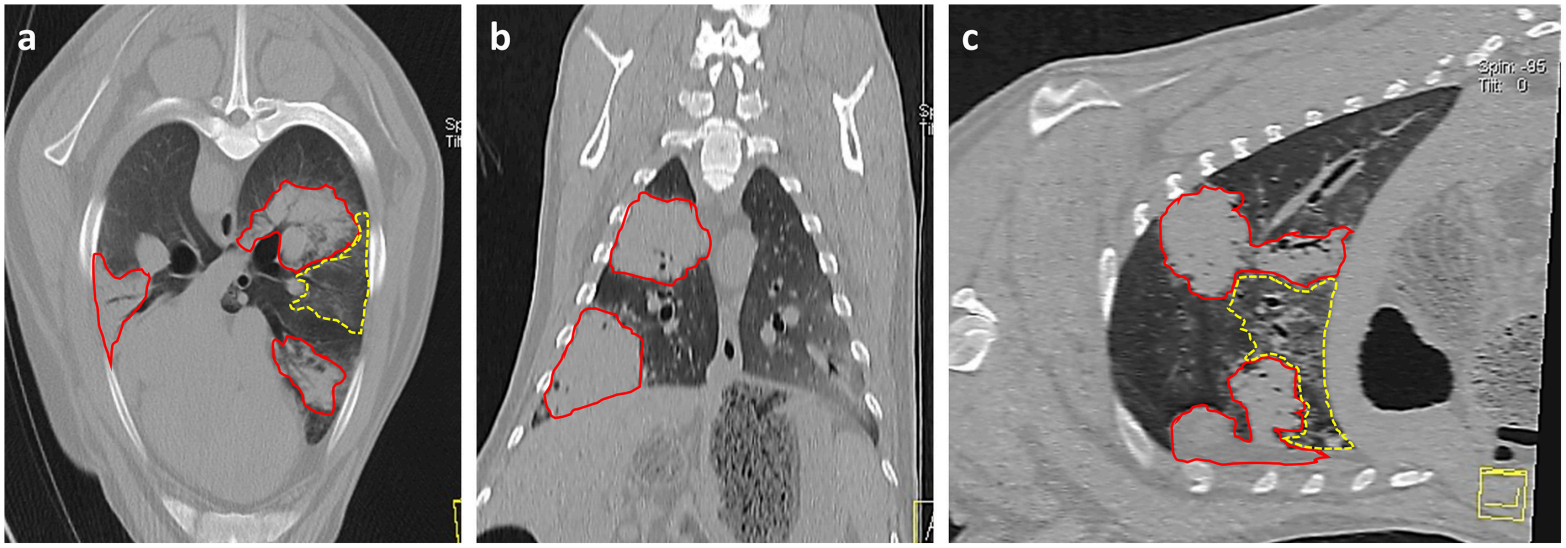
Thoracic CT images of OPA tumors. (a) Axial, (b) Coronal, and (c) Sagittal planes. Three large areas of increased radiopacity are seen within the lung lobes consistent with advanced OPA tumors (outlined in red). One lesion is present within the dorsal region of the left cranial lung lobe with a further lesion in the ventral region of the left caudal lung lobe. A smaller lesion is present within the right caudal lung lobe. A patchy and hazy area of increased opacity (ground glass appearance), with preservation of bronchial and vascular patterns, is present (outlined in yellow) between the two tumors in the left lung lobes. This area is consistent with regions of neoplastic foci or a secondary pneumonia.
